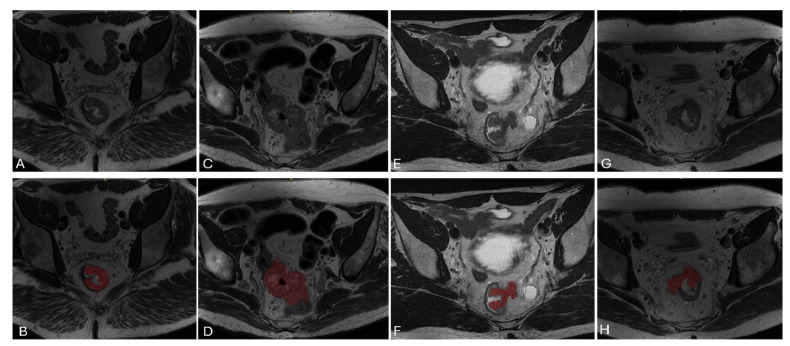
Representative examples of tumor segmentation. Native oblique axial T2-weighted images are shown in the upper row (A,C,E,G), with the corresponding segmentation red overlays presented in the lower row (B,D,F,H).
